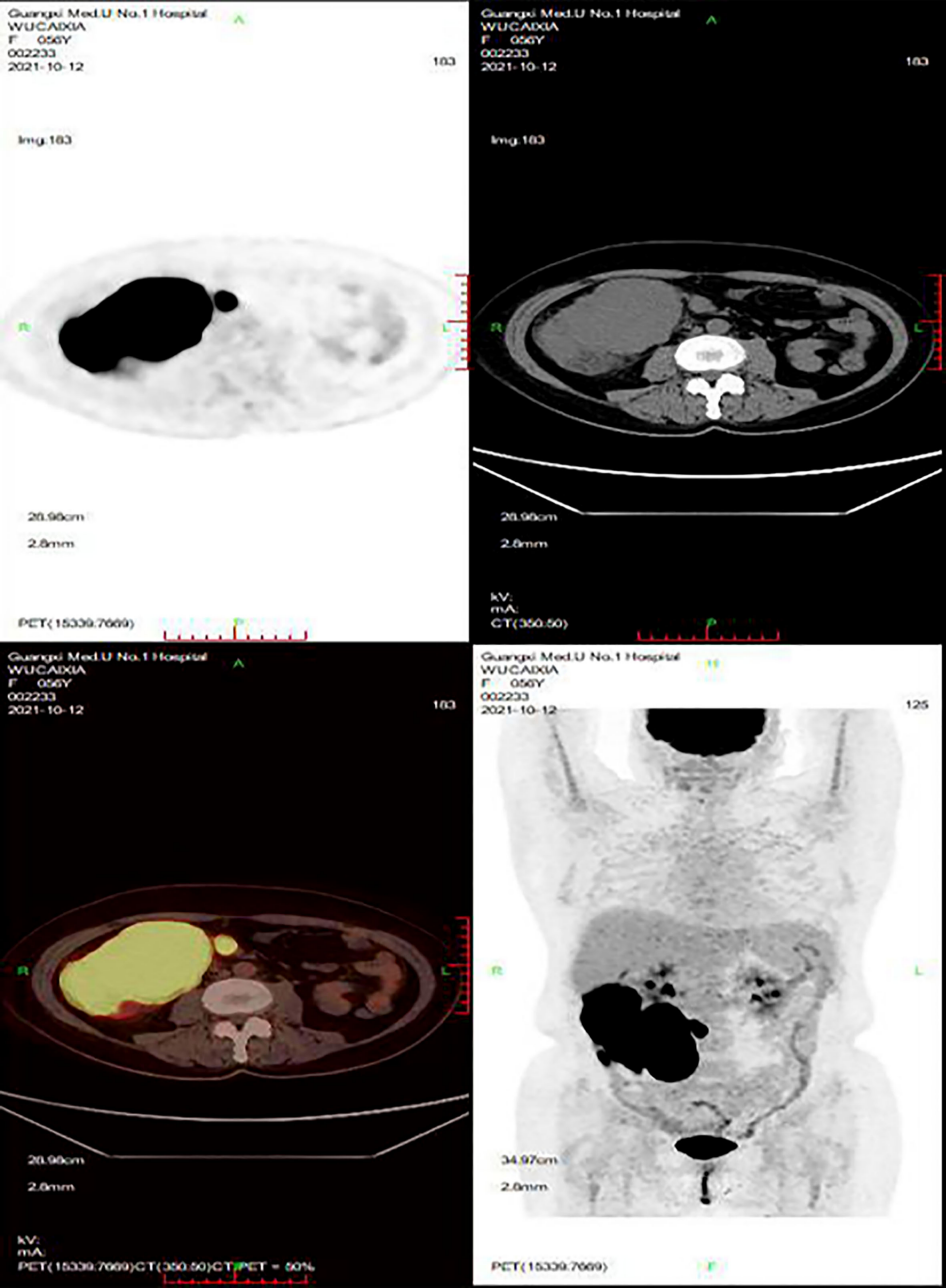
PET/CT. After lymphoma chemotherapy: (A) The lesions in the ileocecal region, the colon, and the mesentery were more advanced than before. (B) The newly developed right anterior lymph node lesions of the diaphragm.
